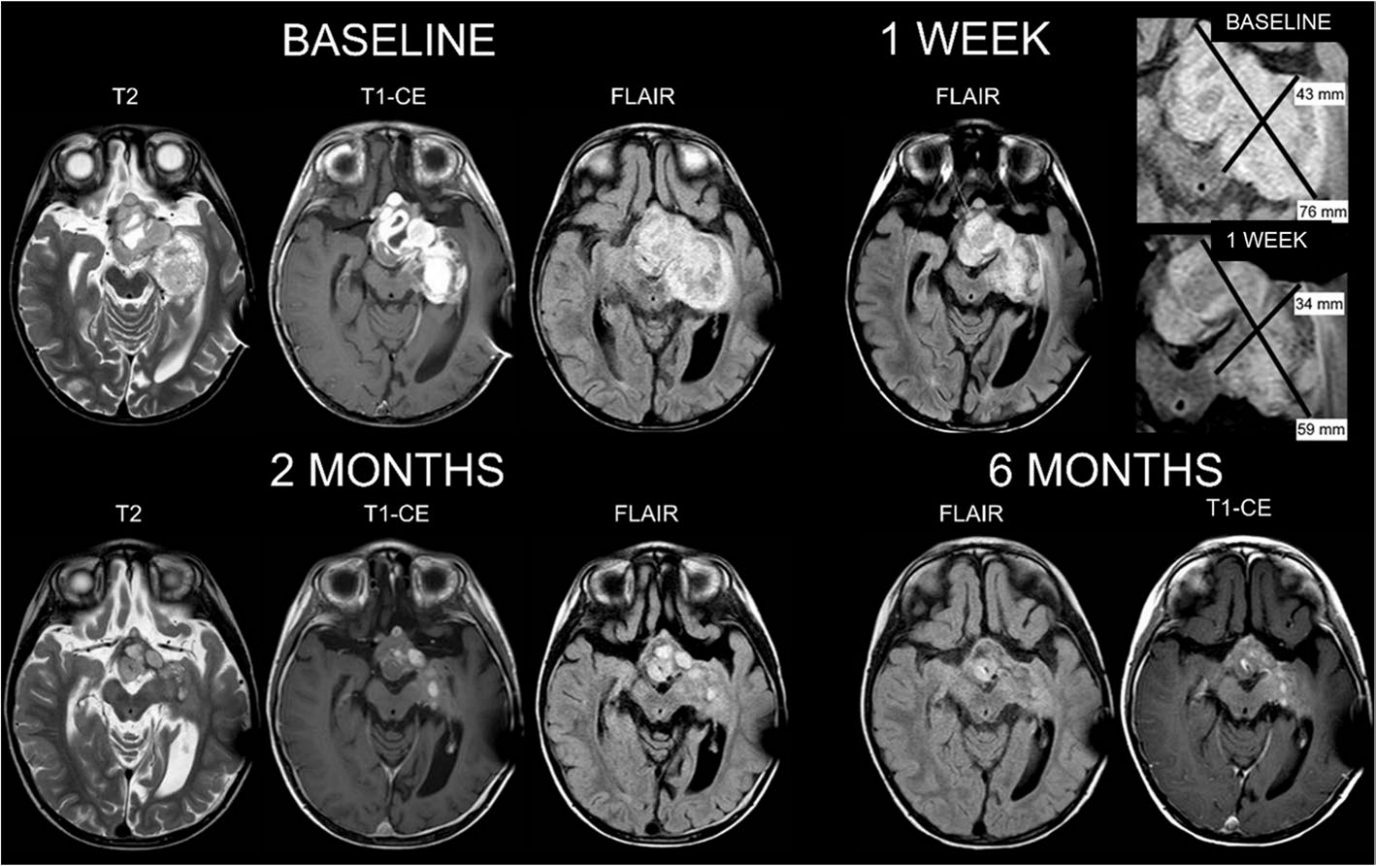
Brain MRI of the 3-year-old boy with pilocytic astrocytoma. Baseline MR and after 2 months in T2-weighted, contrast-enhanced T1-weighted, and FLAIR (FLuid Attenuated Inversion Recovery) show a 61% shrinkage of the mass. MRI at 1 week of therapy (FLAIR) shows a 38% reduction (see small frames, main diameters are reported in millimeters)
